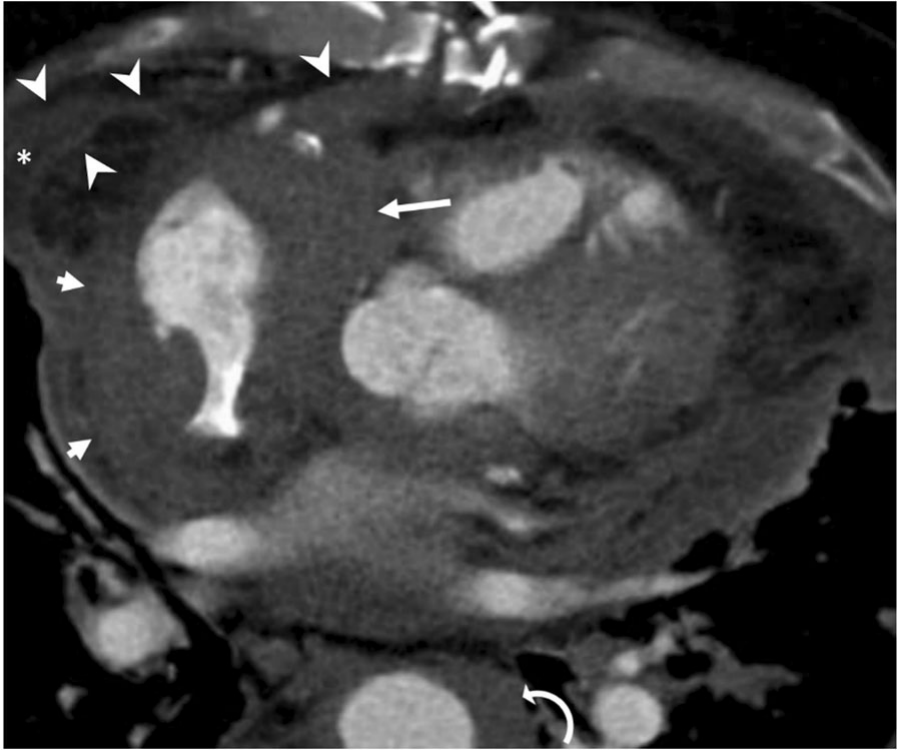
A 61-year-old man with Erdheim-Chester disease. Axial reformatted contrast-enhanced CT image demonstrates lobulated soft tissue densities with pseudo-tumor appearance involving the right atrium (short arrows), right atrioventricular groove (long arrow), and the aortic wall (curved arrow). Pericardial (asterisk) effusion and thickening (arrowheads) are also seen
